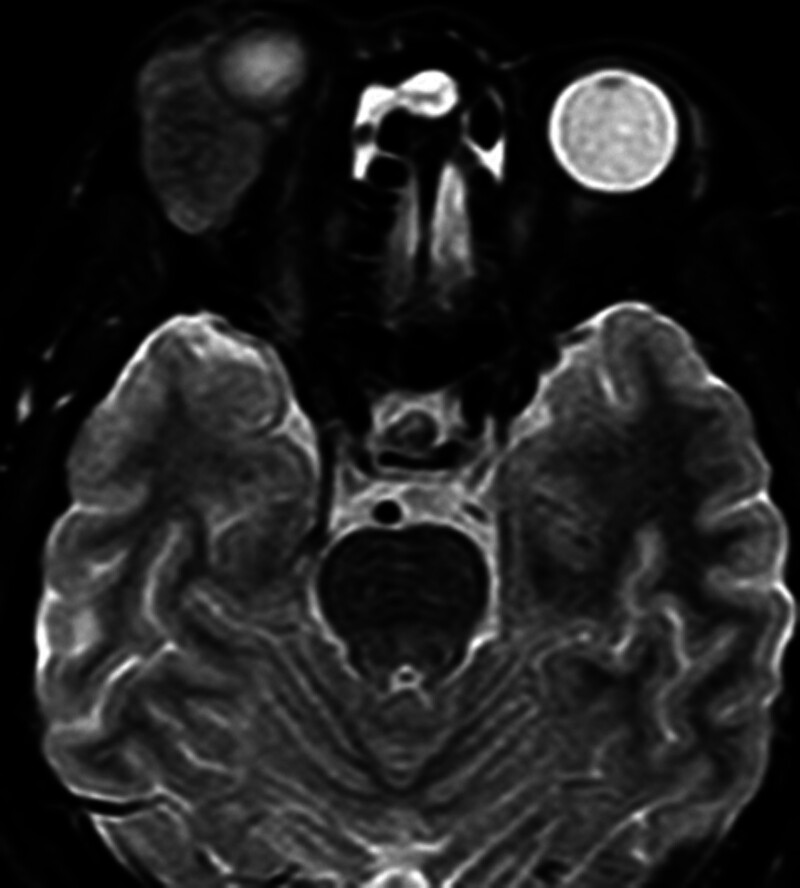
MRI suggested that the right upper quadrant of the orbit (outside the muscle cone) had an abnormal signal. T1WI was isosignal, and the enhancement was more uniform and obvious. MRI = magnetic resonance imaging, T1WI = T1 weighted image.
